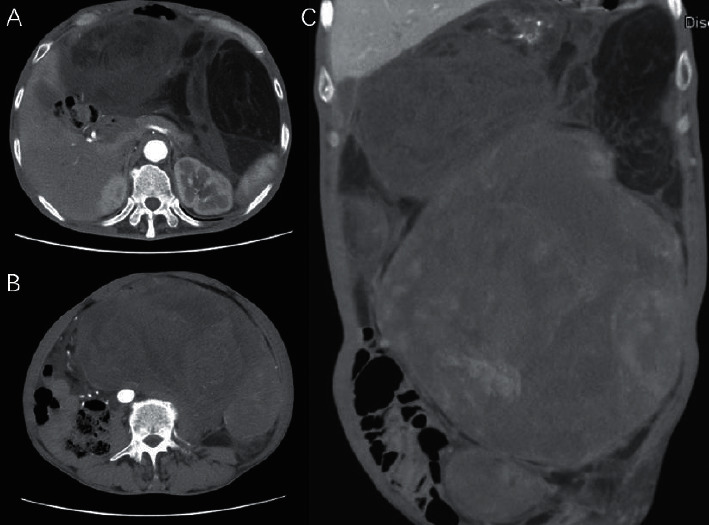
CT scan of retroperitoneal MLS patients. (a) Huge retroperitoneal MLS visible at kidney level. (b) Huge retroperitoneal MLS visible at colon level. (c) Huge retroperitoneal MLS, coronal view.
